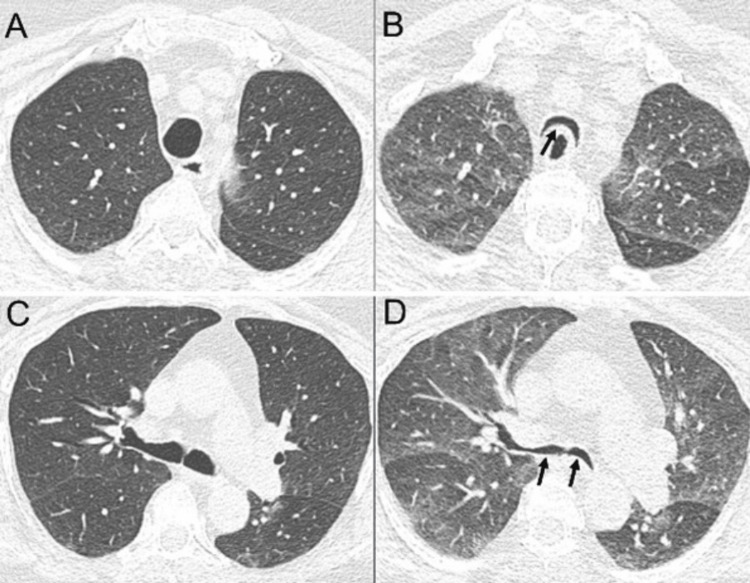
Excessive dynamic airway collapse. Axial CT images during inspiration (A and C) and expiration (B and D) demonstrate excessive dynamic collapse of the trachea and main bronchi (arrows), along with patchy expiratory air trapping. Notably, there is excessive anterior bowing of the posterior tracheal wall during expiration resulting in a crescent-shaped trachea.
